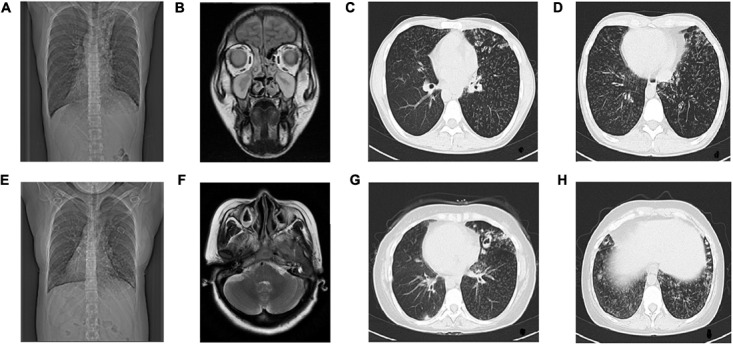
Radiological examination of Proband and Proband’s Sister. The radiological examination of Proband (II-5), (A) CT showed situs inversus. (B) Coronal view of paranasal sinus MRI shows bilateral maxillary sinusitis and ethmoid sinusitis. (C,D) HRCT of chest shows bronchitis and bronchiolitis. Bronchiectasia involves in left lobes. Centrilobular nodules and tree-in-bud sign distribute diffusely in both lobes.The radiological examination of Proband’s sister (II-1), (E) CT showed situs inversus, (F) Transverse view of sinus MRI showed bilateral maxillary sinusitis, (G,H) HRCT of chest showed bronchitis and bronchiolitis, ring-shaped or ductal opacities bronchiectasia involves in both lobes. Secretion obstruction can be seen in some saccular bronchiectasis in the upper left lobes.
